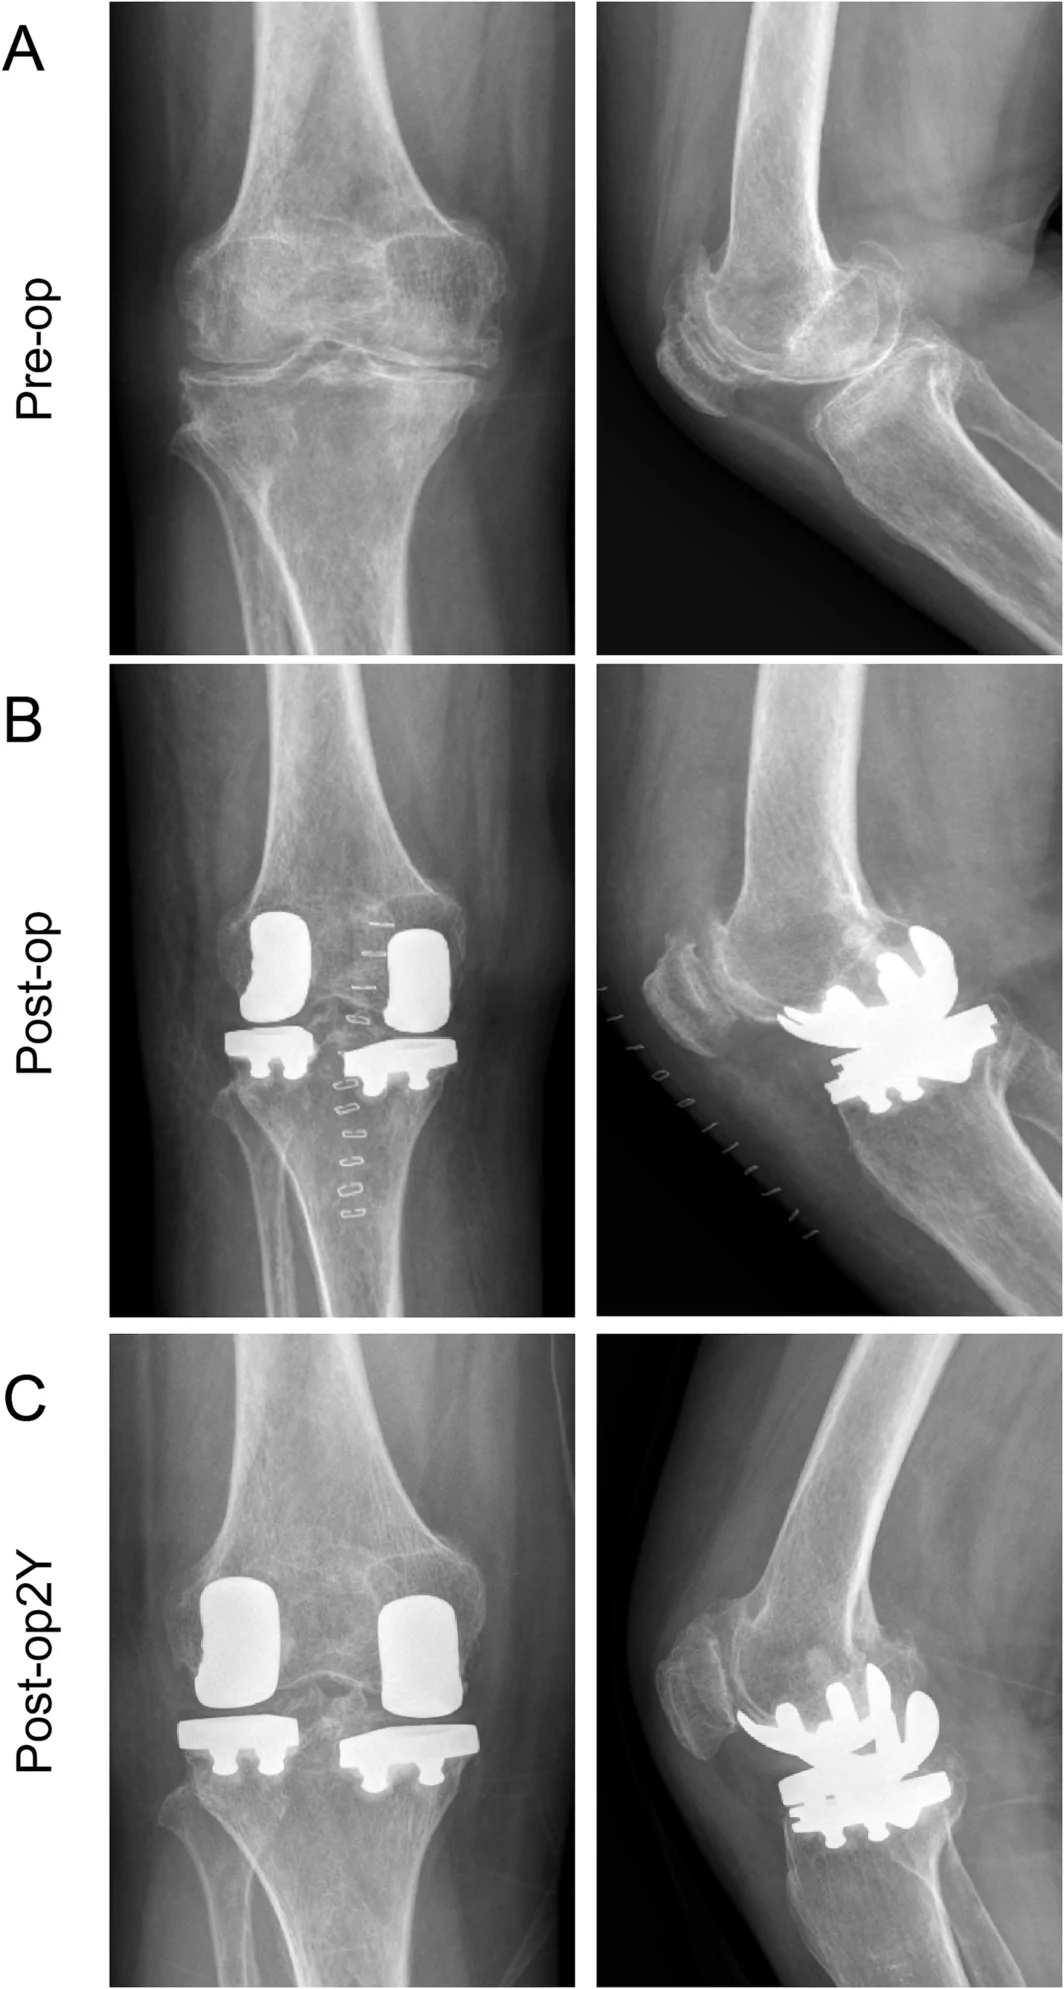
Radiographic comparison of the knee joint before and after bicompartmental unicompartmental knee arthroplasty (bi‐UKA). (a) Preoperative anteroposterior (AP) and lateral view radiographs of the knee show significant narrowing of both the medial and lateral joint spaces, irregularity of the articular surfaces, and osteophyte formation, indicative of bicompartmental osteoarthritic changes. (b) Immediate postoperative AP and lateral view radiographs demonstrate proper implantation of the medial and lateral unicompartmental prostheses, with good alignment and congruency between the components and joint surfaces. (c) AP and lateral view radiographs at 2 years postoperatively reveal stable implant positioning, preserved joint space, and no signs of loosening, migration, or periprosthetic complications. The bony margins show no evidence of osteolysis or bone resorption, indicating a favorable mid‐term radiographic outcome.
